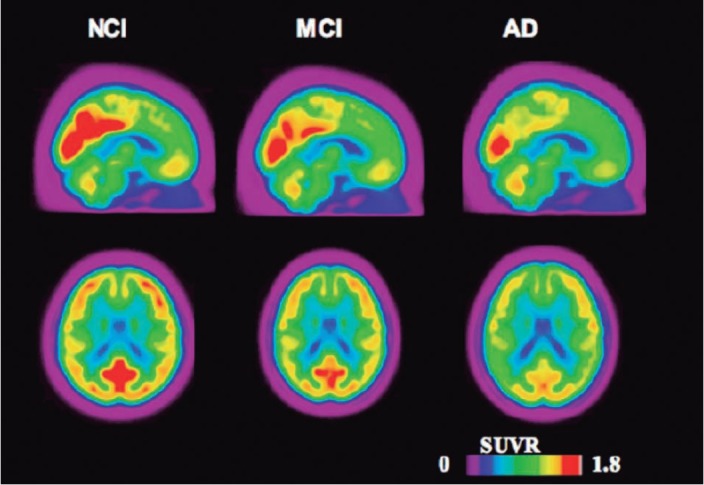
Representative PET [^18^F]FDG images acquired from a total of 103 individuals with normal cognition (NCI, N= 17), mild cognitive impairment (MCI, N=52) and dementia due to AD (AD, N= 27) obtained from a total of 103 structural MRI and [^18^F]FDG scans from the Alzheimer's Disease Neuroimage Initiative (ADNI) database. The hot color scale represents the magnitude of [^18^F]FDG standardized uptake value ratio (SUVR), proportional to glucose uptake. Note lower [^18^F]FDG SUVRs in MCI and AD as compared to controls. High SUVRs are particularly reduced in the posterior cingulate, precuneus and prefrontal (medial and dorsolateral) cortices. Imaging Methods: MRI images were corrected for non-linearity, classified (white, grey matter and CSF) and automatically segmented into cortical regions. Standard uptake value ratio (SUVR) images were calculated in PET native space using the cerebellum as a reference image. Images were subsequently resampled into the standard stereotaxic space and averaged using minctools.
